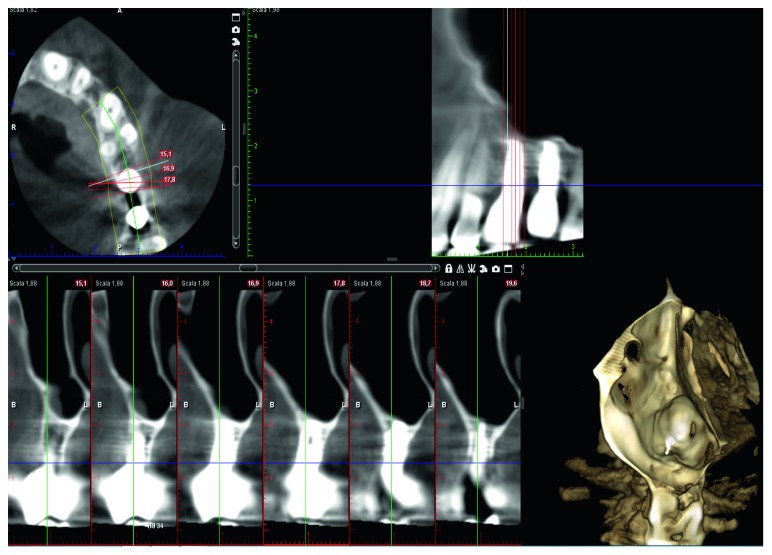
Implant site #25 CT scan at 4 years.
